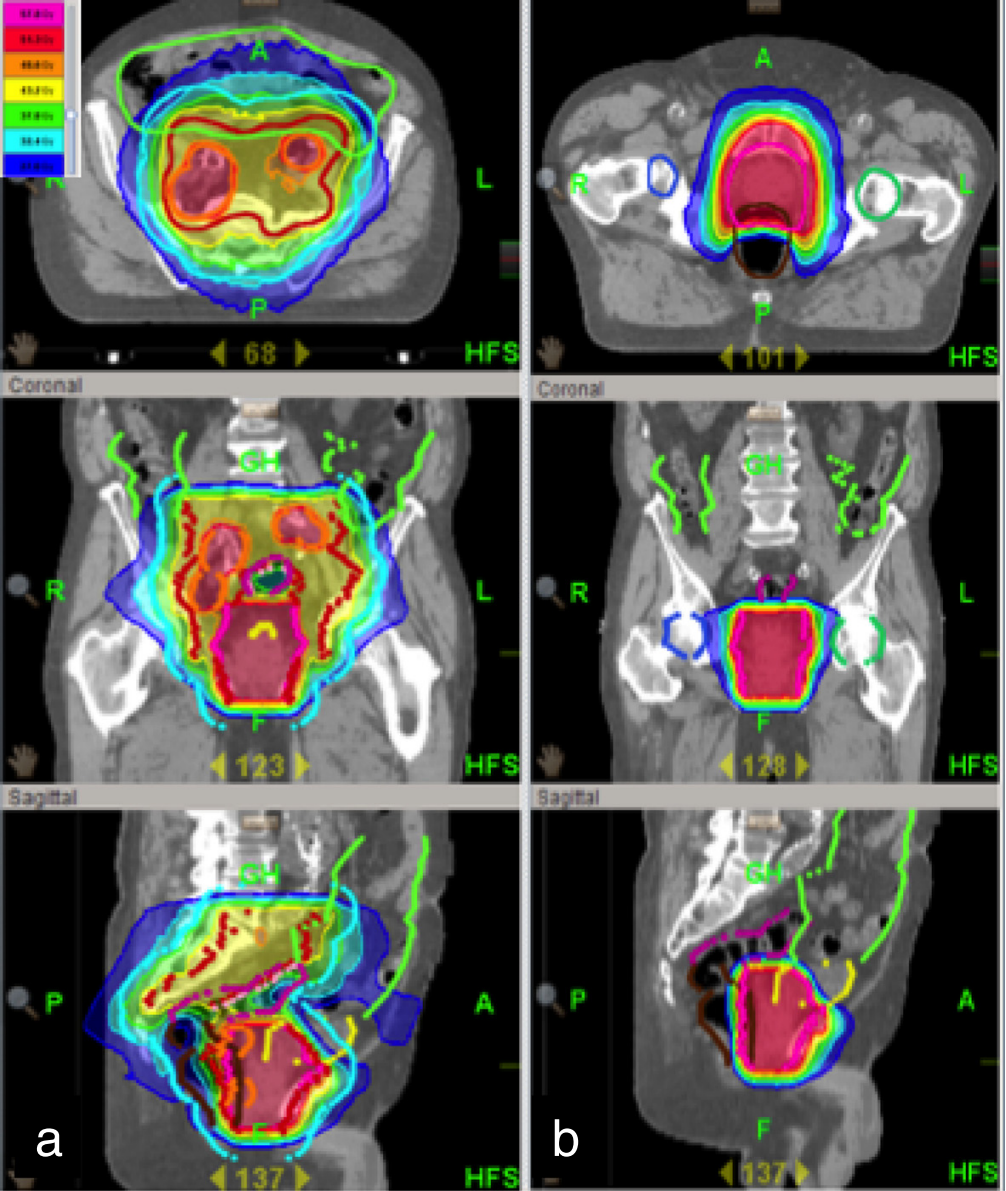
Treatment plan comparison. Basic treatment plan (a), including lymph node irradiation up to 45 Gy à 1.8 Gy. Based on the information obtained by ^68^Ga-PSMA-PET imaging, PET-positive nodes receive a simultaneous integrated boost to 54 Gy à 2.17 Gy. In comparison with Fig. 4a an example of the dose distribution (b) without the knowledge through the ^68^Ga-PSMA-PET; the lymph node involvement would not have been suspected and therefore only the prostate itself would have been treated
